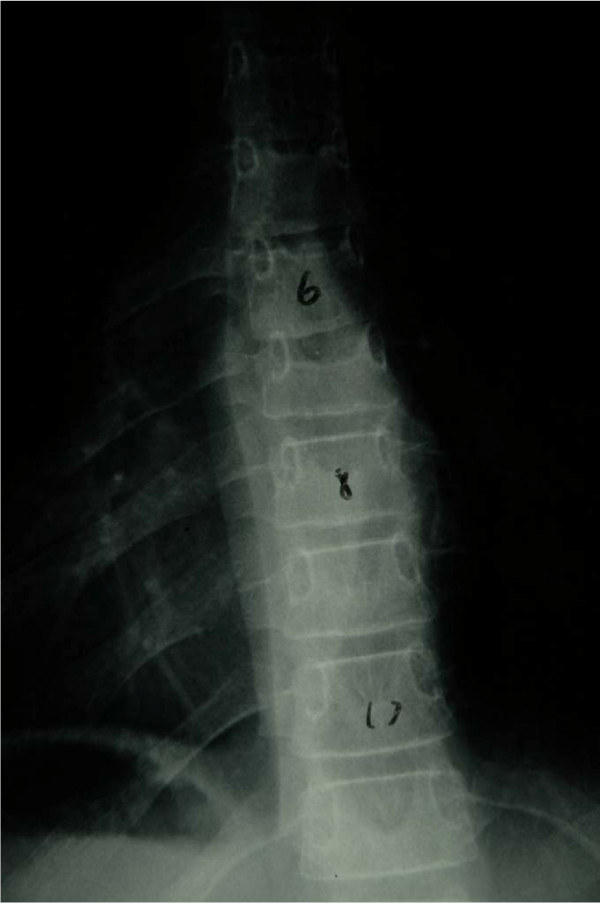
Spine radiograph demonstrating an osteoporosis-related T7 vertebral fracture.
